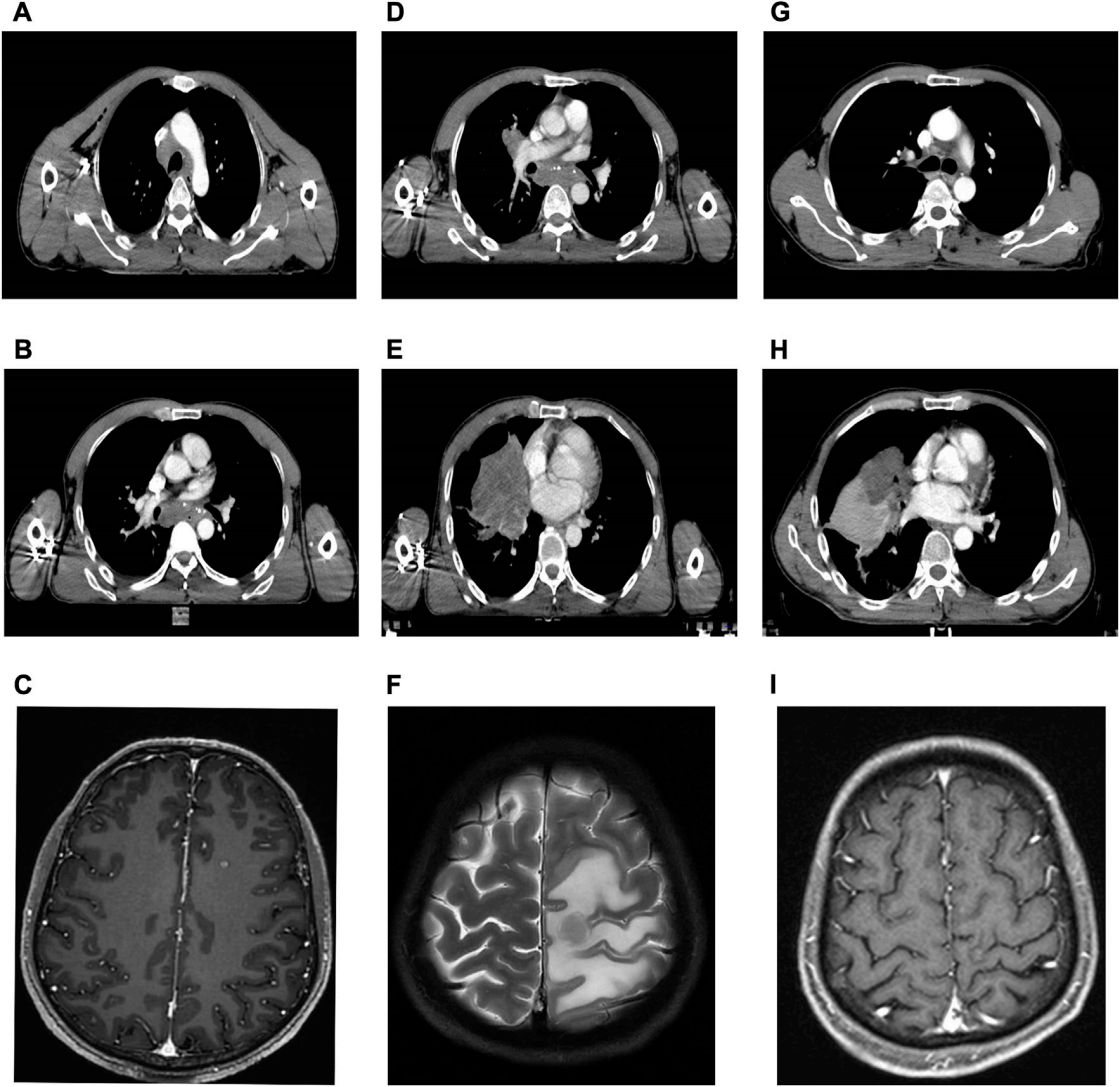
Serial CT and MRI of the case. (A,B) Baseline CT at diagnosis of the lung. (C) Baseline CT at diagnosis of the brain metastases. (D,E) CT after 3 months of osimertinib therapy with progressive disease of the lung. (F) MRI after 3 months of osimertinib therapy with progressive disease of the brain metastases with encephaledema. (G,H) CT after 3 months of alectinib therapy with a partial response of the lung. (I) MRI after 3 months of alectinib therapy and radiotherapy with a partial response of the brain metastases.
